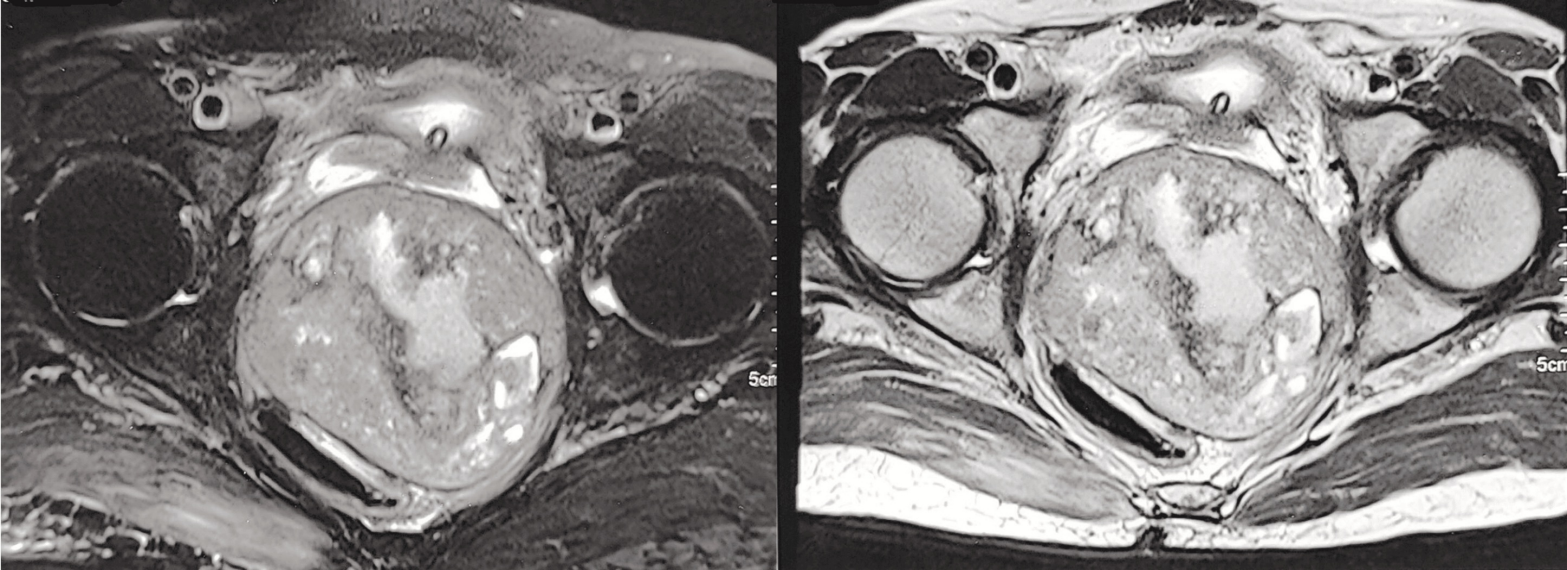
MRI pelvis showing solid cystic mass (88x87x90 mm) lesion in lesser pelvic cavity that was hyperintense in T2 weighted images in T1 weighted images, hyperintense area- compressing rectum towards right with narrowing of its lumen with focal loss of intervening fat planes is seen
